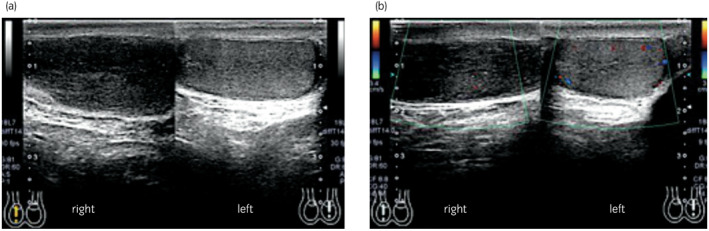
Ultrasonography indicates a homogeneous internal hypoechoic lesion in the right testis (a). Color doppler ultrasonography reveals decreased blood flow in the lesion (b).
